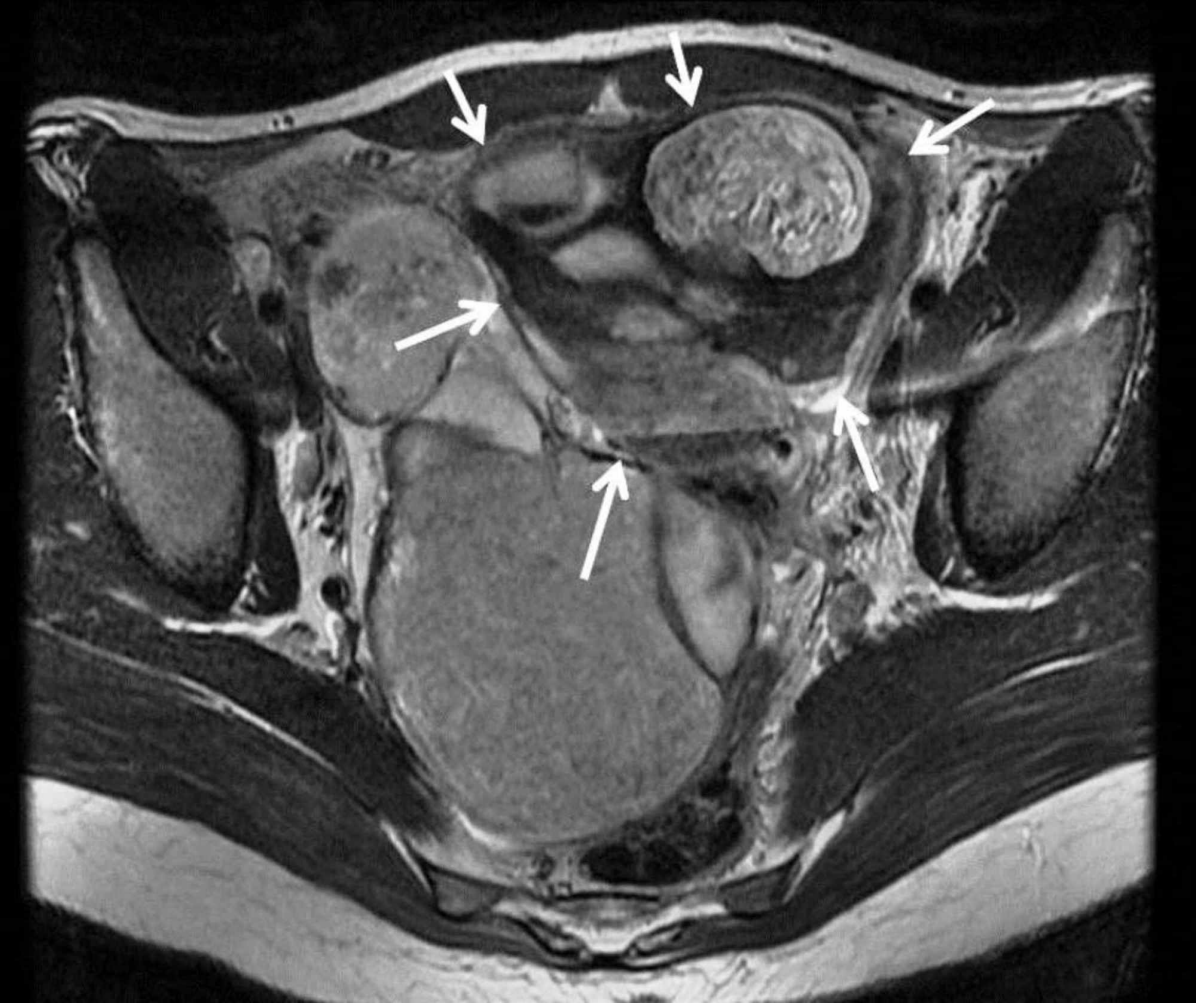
Magnetic resonance imaging scan of the pelvis View demonstrating a large thin-walled dermoid cyst of the left ovary
